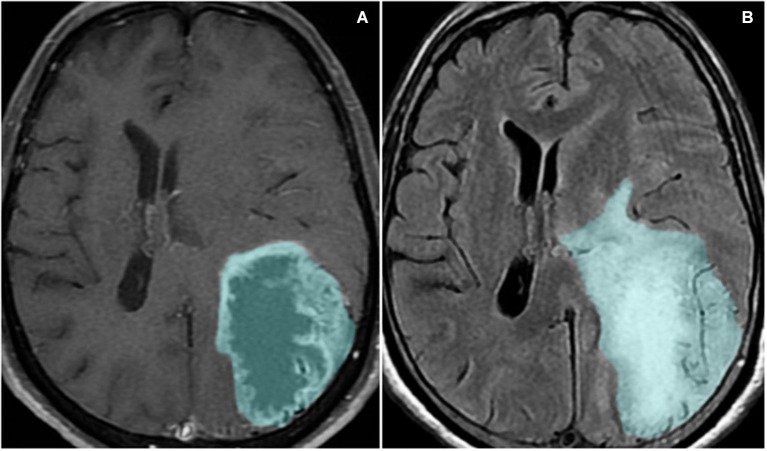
Magnetic resonance imaging sequences T1-weighted with contrast (A) and FLAIR (B) show a left parieto-occipital mass with high signal on FLAIR and a surrounding area of vasogenic edema/tumor infiltration. The lesion presents peripheral heterogeneous contrast enhancement, representing the disruption of the blood-brain barrier.
